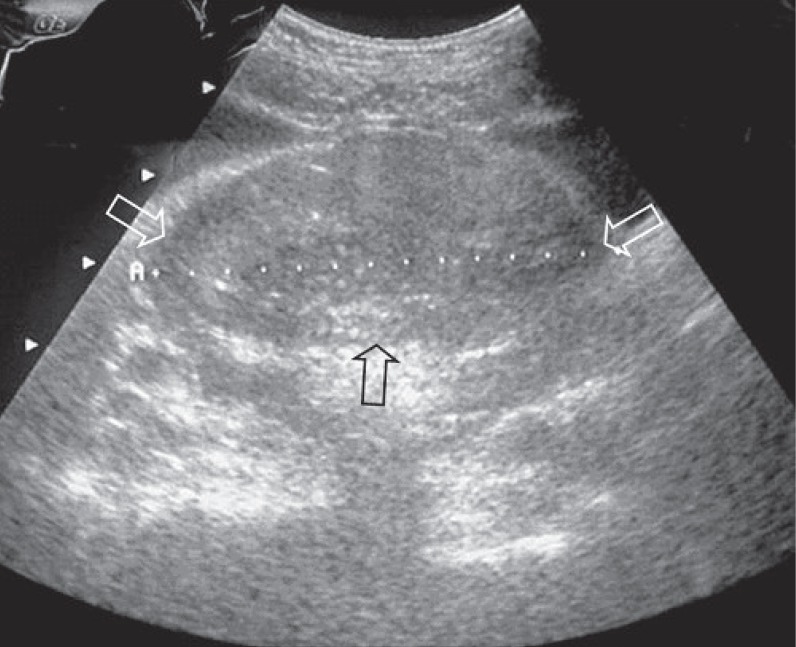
Pelvic ultrasound shows a 8 cm solid,heterogeneous, pelvic mass (arrow) to the posterior side of the urinary bladder.
